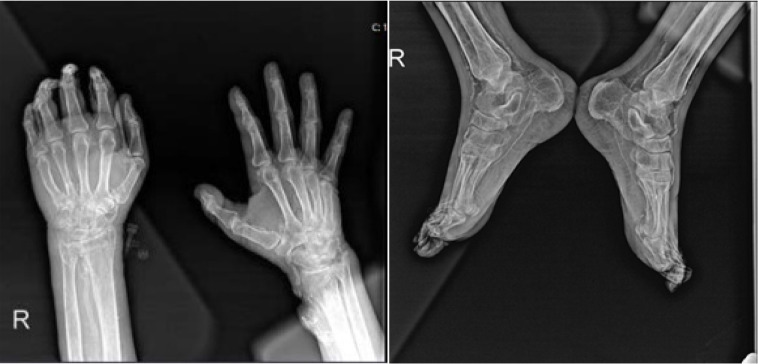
Hand and feet X-rays showing calcifications (shown with arrows).
